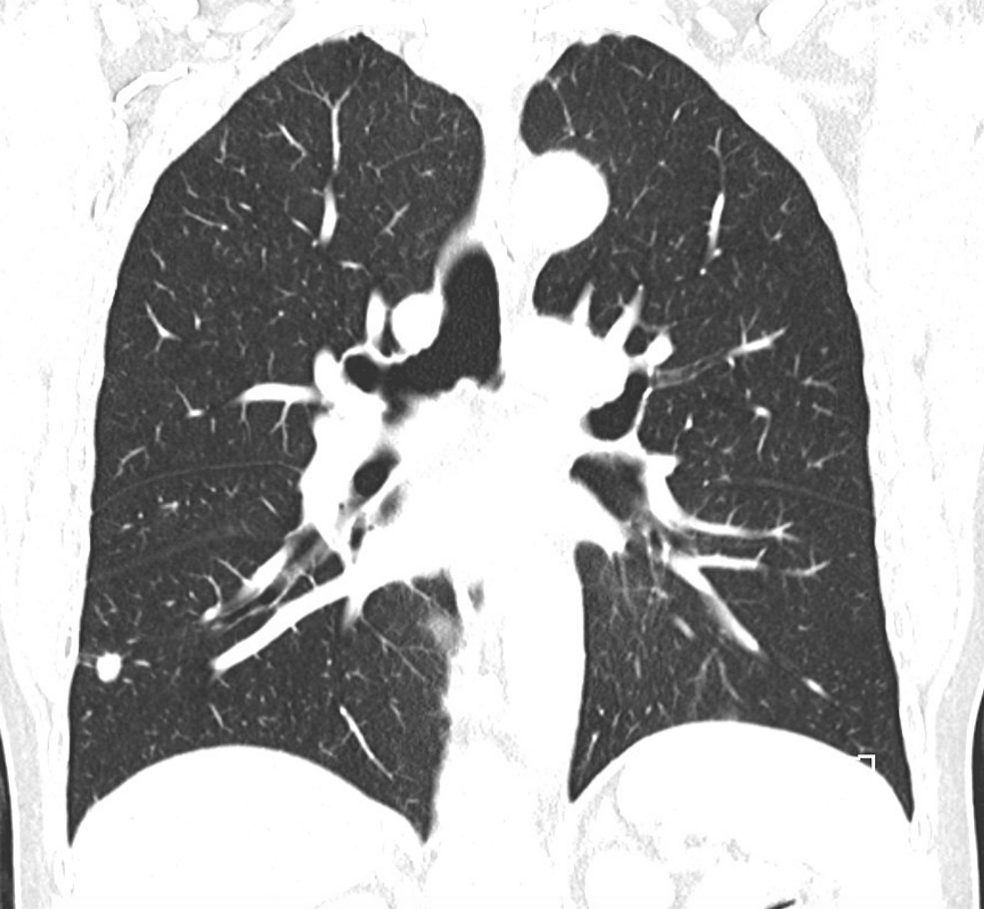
CT coronal section depicting right sided lung mass that was confirmed as a stage IA2 primary, epidermal growth factor receptor (EGFR) positive non-small cell lung cancer on biopsy
